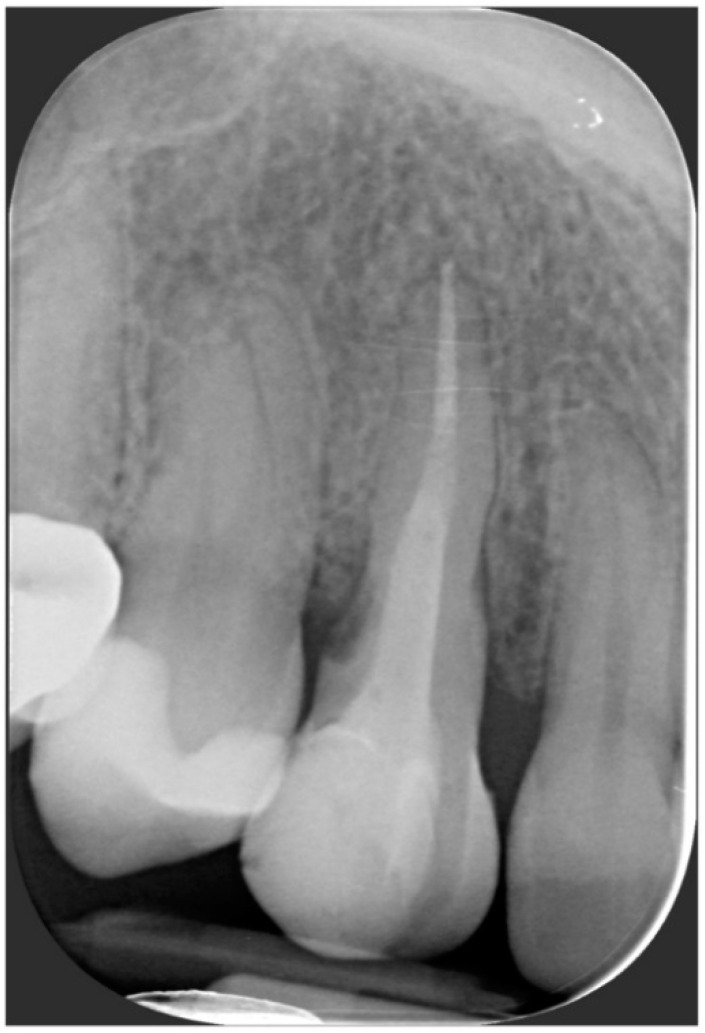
Radiographic evaluation revealing internal root resorption.
